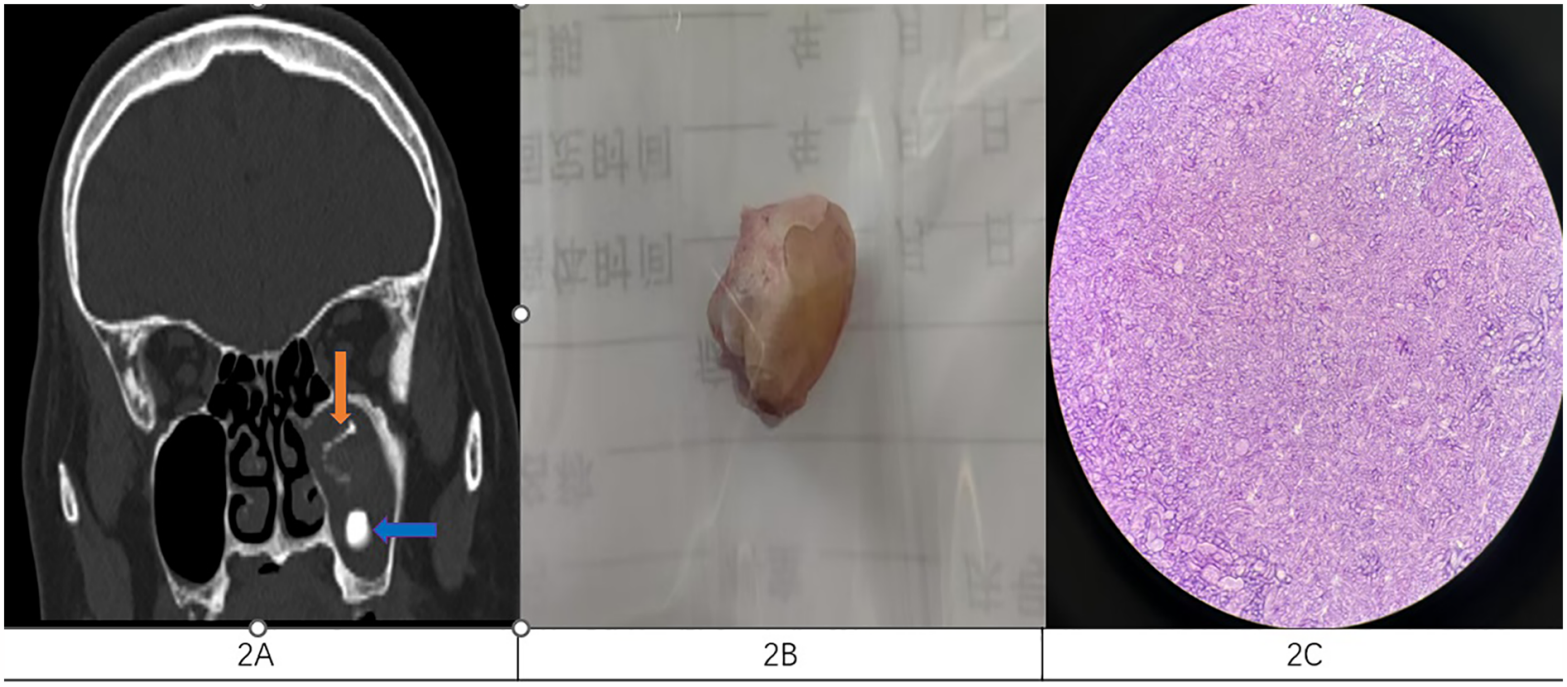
(A) Coronal CT scan of the sinuses showed the presence of both lesions orange for fungal infection and blue for ectopic teeth. (B) The ectopic tooth was removed intraoperatively. (C) Pathological smears showing fungi.
